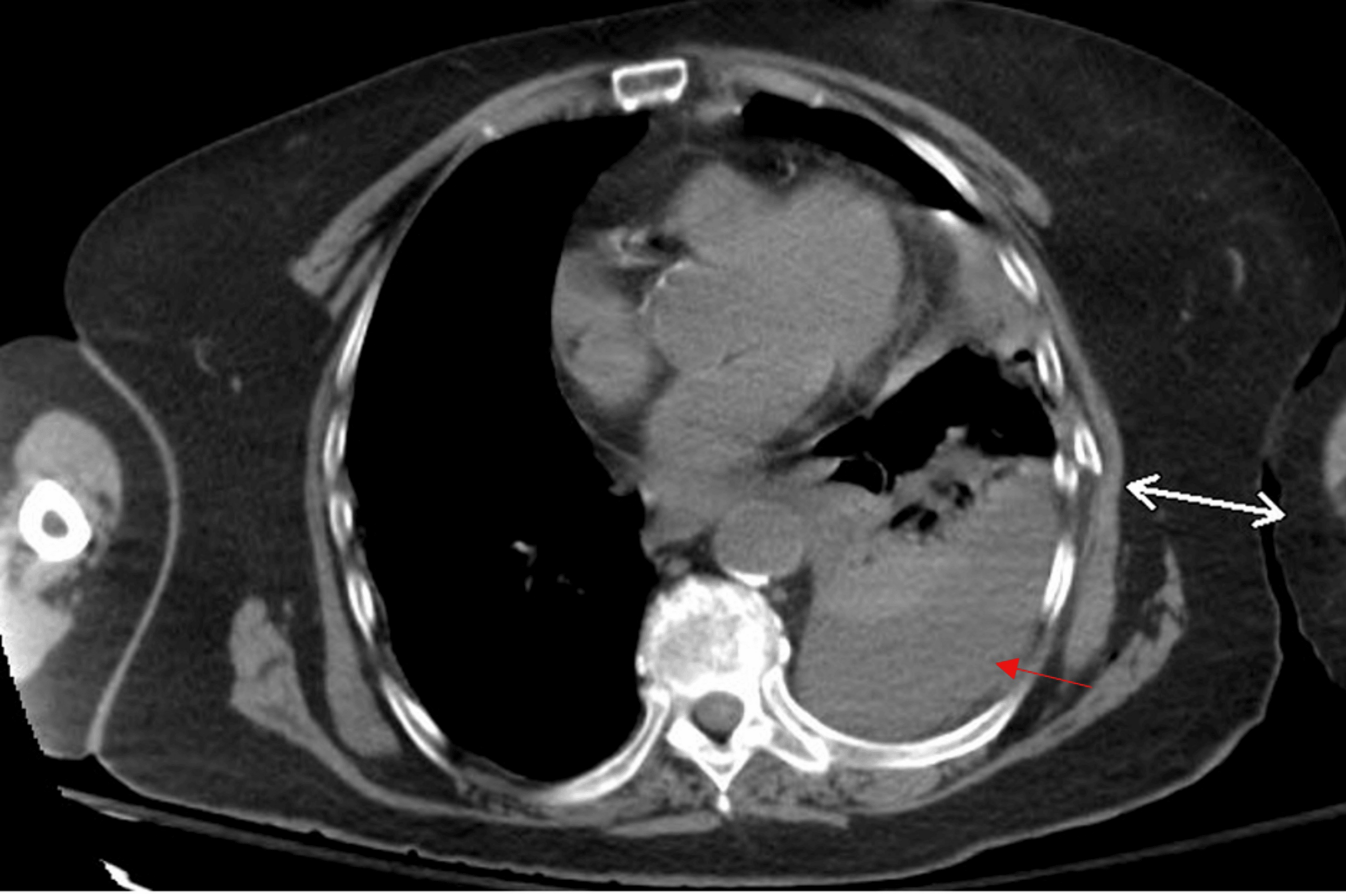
Axial non-contrast CT chest scan. The image shows a left-sided hemothorax (red arrow). A bidirectional arrow highlights the left rib fracture.
